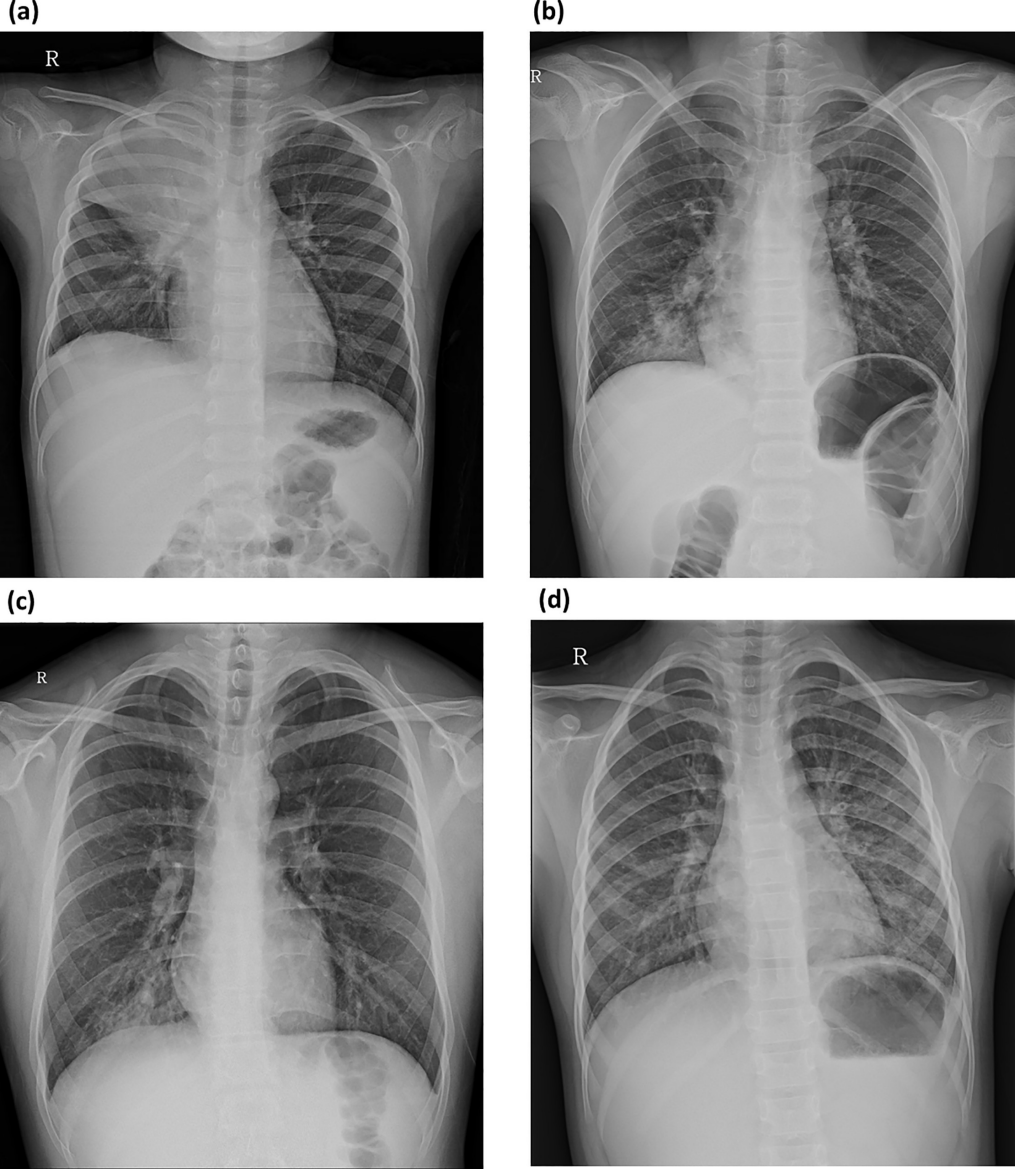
Categorization of the radiographic findings of *M*. *pneumoniae* pneumonia. (a)
